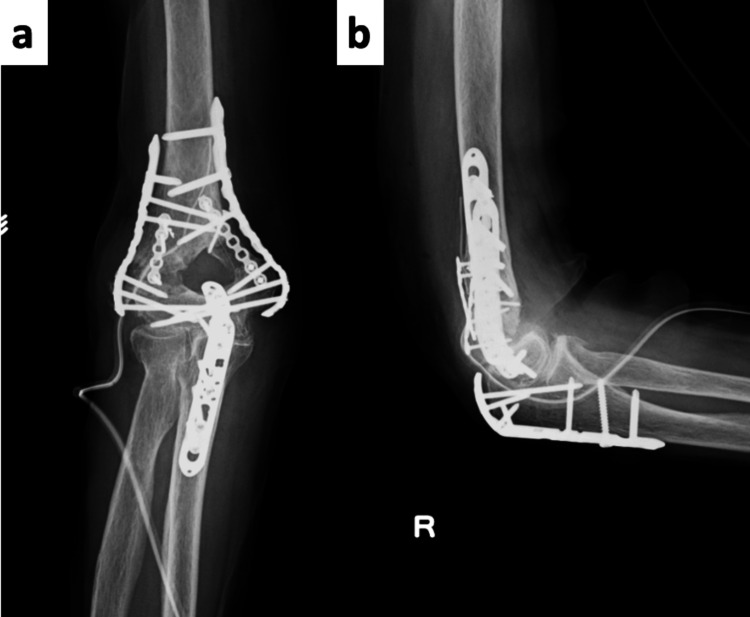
Postoperative Plain Radiographs (a) Anteroposterior (AP) view; (b) lateral view. Postoperative images demonstrate good reduction and stable fixation.
